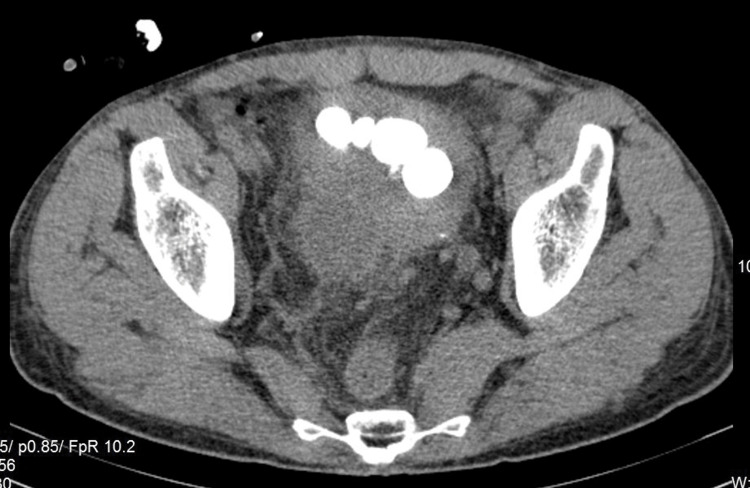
Axial plane of contrast CT scan showing multiple vesical calculi. CT, computed tomography
